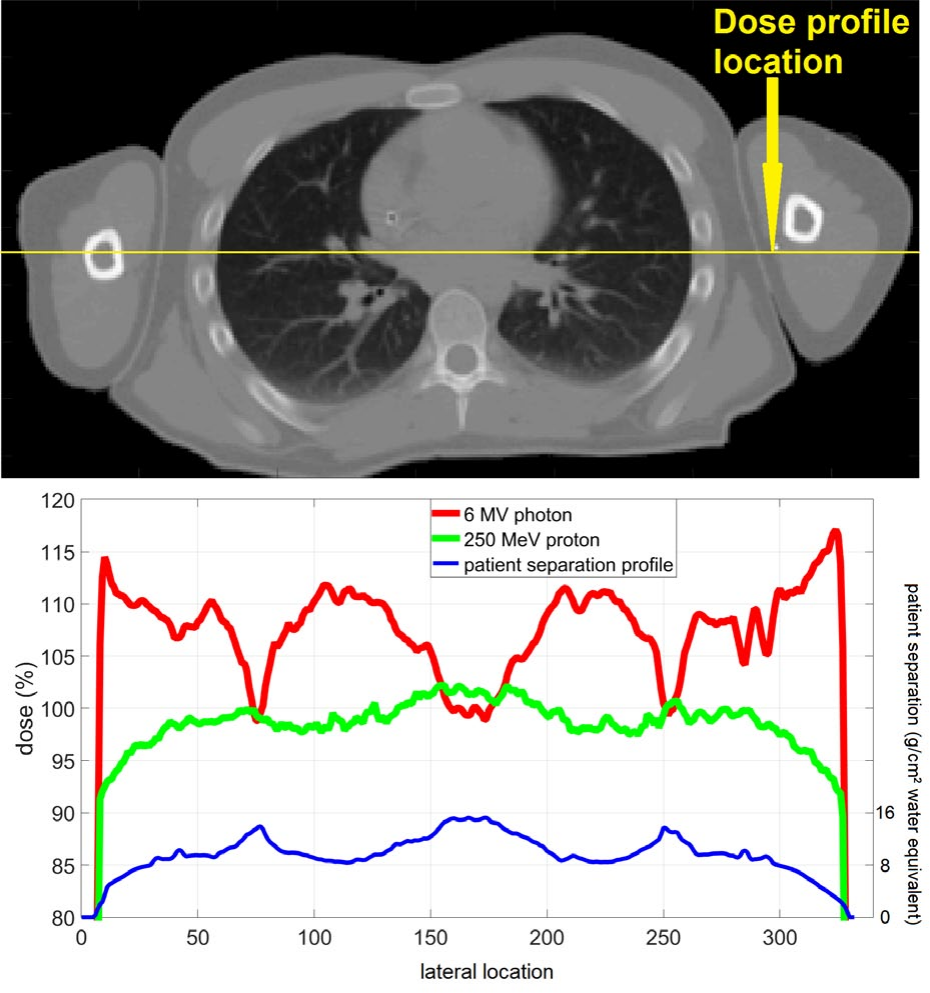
A CT slice showing mediastinum region (top) and the dose profiles of 250 MeV proton and 6MV photon (bottom). The location of the profile was marked in the CT image. The patient separation profile along the marked line was also plotted as a reference in the bottom graph.
